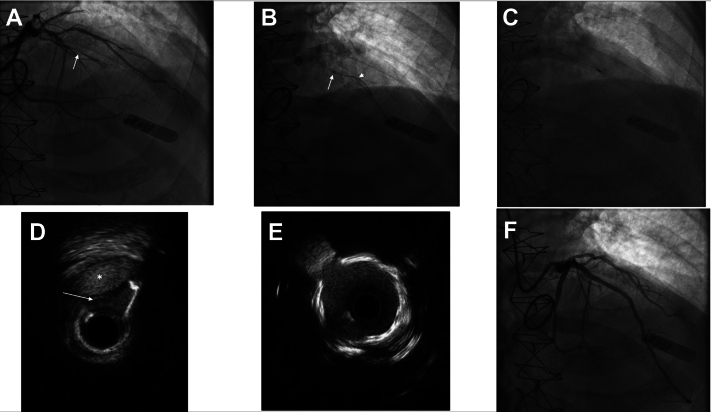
Extraplaque laser to assist in crossing occlusionsstepwise approach. (A) Chronic total occlusion (CTO) of mid left anterior descending (arrow) with bridging collateral providing distal flow in conjunction with retrograde collaterals (not shown). (B) A 0.9-mm Excimer laser (Philips) shown at the point of subintimal entry over the Gladius Mongo wire (Asahi Intecc), with continued ablation until just distal to the CTO segment (arrowhead). (C) A 1.5-mm rotational atherectomy burr (ROTAPRO, Boston Scientific) taken through the lesion. (D) Intravascular ultrasound following the extraplaque laser to assist in crossing occlusion method with subsequent rotational atherectomy showing subintimal space used in extraplaque laser to assist in crossing occlusion (asterisk) and disruption of circumferential calcification adjacent to the dissection plane (arrow). (E) Post–percutaneous coronary intervention intravascular ultrasound at the site of CTO showing full stent expansion and apposition. (F) Angiography after percutaneous coronary intervention showing no residual lesion.
